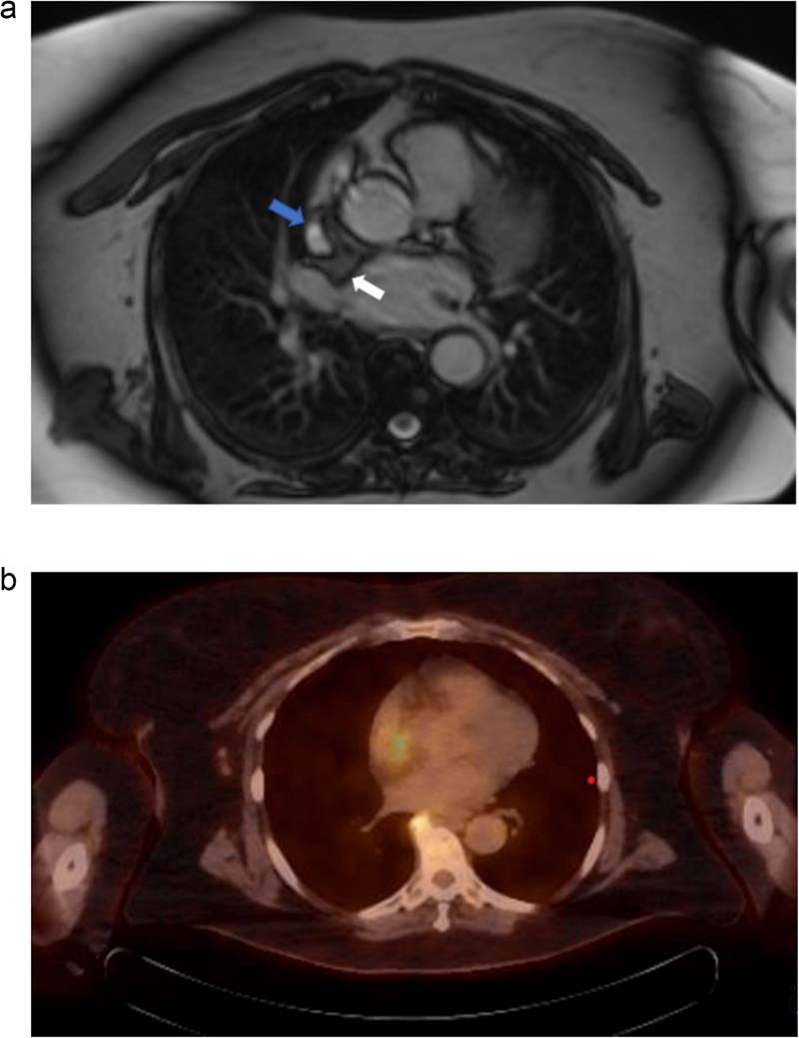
(a) Cardiac MRI. Mass is represented by the arrow on the right. The left arrow demonstrates the SVC. (b) PET/CT. Uptake is demonstrated in the right atrium. Uptake near the spine was determined to be artifact and not representative of metastatic disease.
